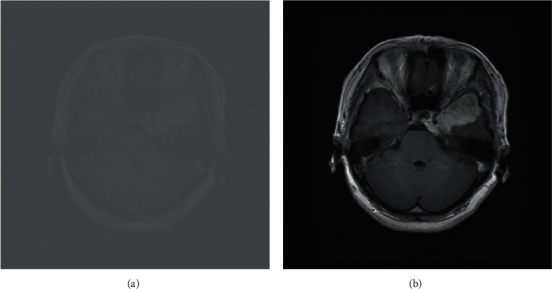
MRI before and after normalization.
